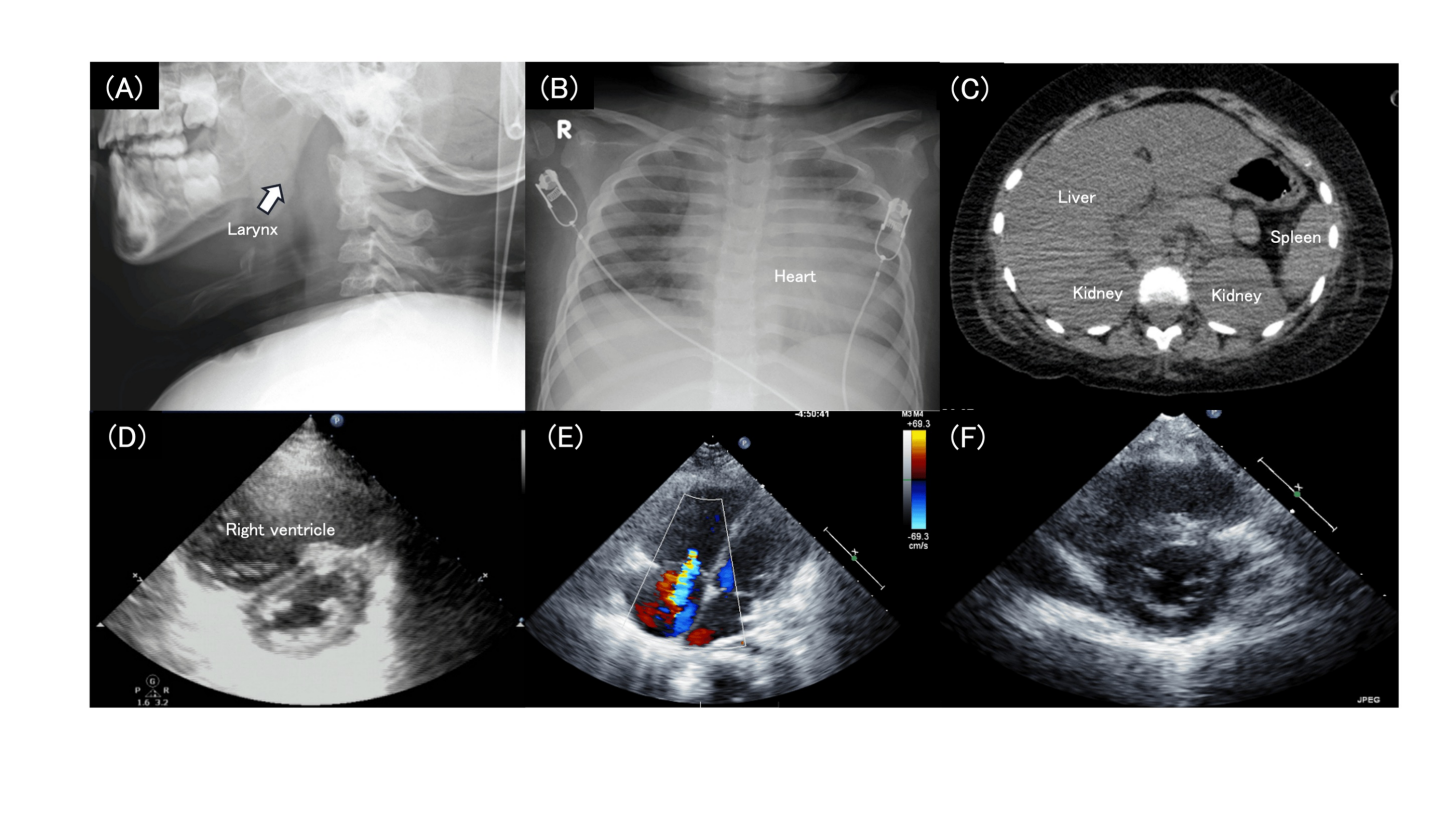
Imaging findings during acute respiratory decompensation (A) Lateral neck radiograph demonstrating narrowing of the upper airway at the laryngeal level, (B) Chest radiograph showing bilateral decreased lung transparency and cardiomegaly with a cardiothoracic ratio of 0.68, (C) Abdominal computed tomography demonstrating hepatomegaly with increased hepatic attenuation, (D, E) Echocardiography at admission demonstrated marked right ventricular hypertrophy resulting in a D-shaped left ventricle. Tricuspid regurgitation was also observed, suggesting the presence of pulmonary hypertension, (F) Follow-up echocardiography one month later showed improvement in right ventricular dilatation and resolution of left ventricular compression.
